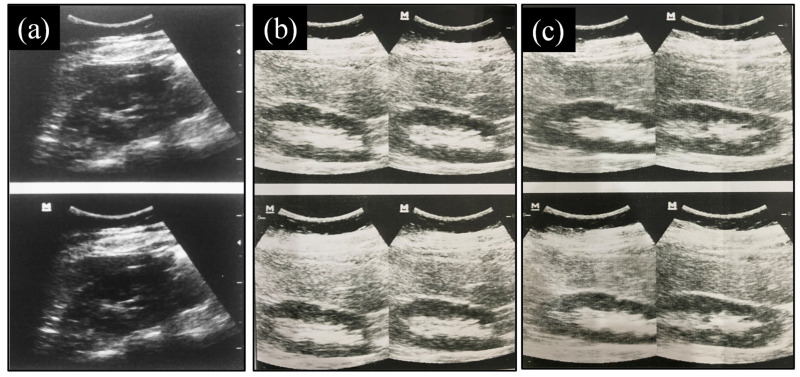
Ultrasound image of the right kidney produced (a) without any conductive medium, (b) when a selected formulation (S1) was applied to the skin, and (c) when a commercial gel (CG) was applied to the skin.
